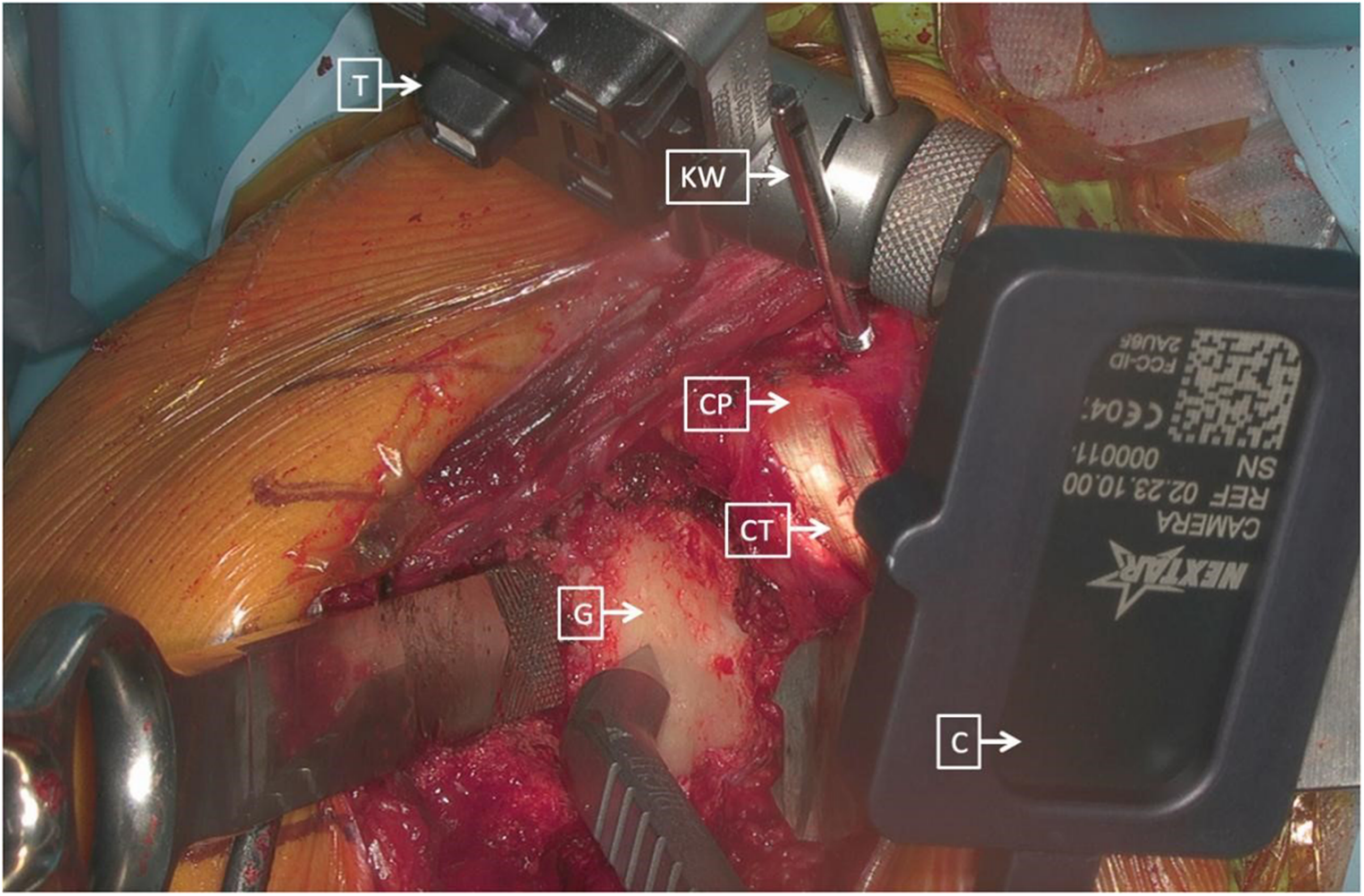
Right shoulder, beach chair position. The tracker (T) was placed and secured on the K-wire (KW) and on the beak (CP), consistently with the cameras (C) that were placed on different instruments (CT, combined with tendons; G, glenoid) (18).
